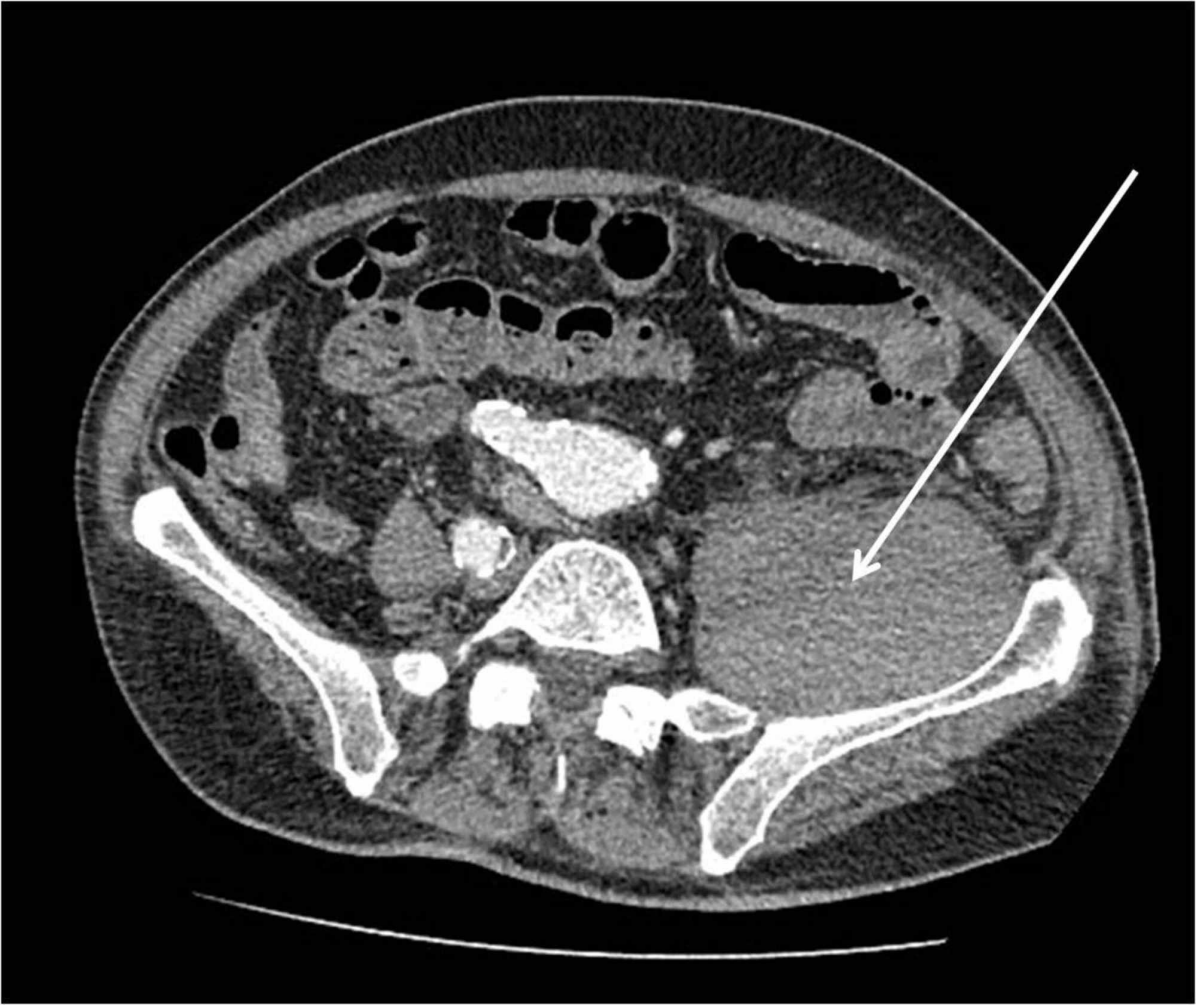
CT scan performed on day 3 post-admission did not reveal any significant change in regards to the size of the haematoma (arrow).
